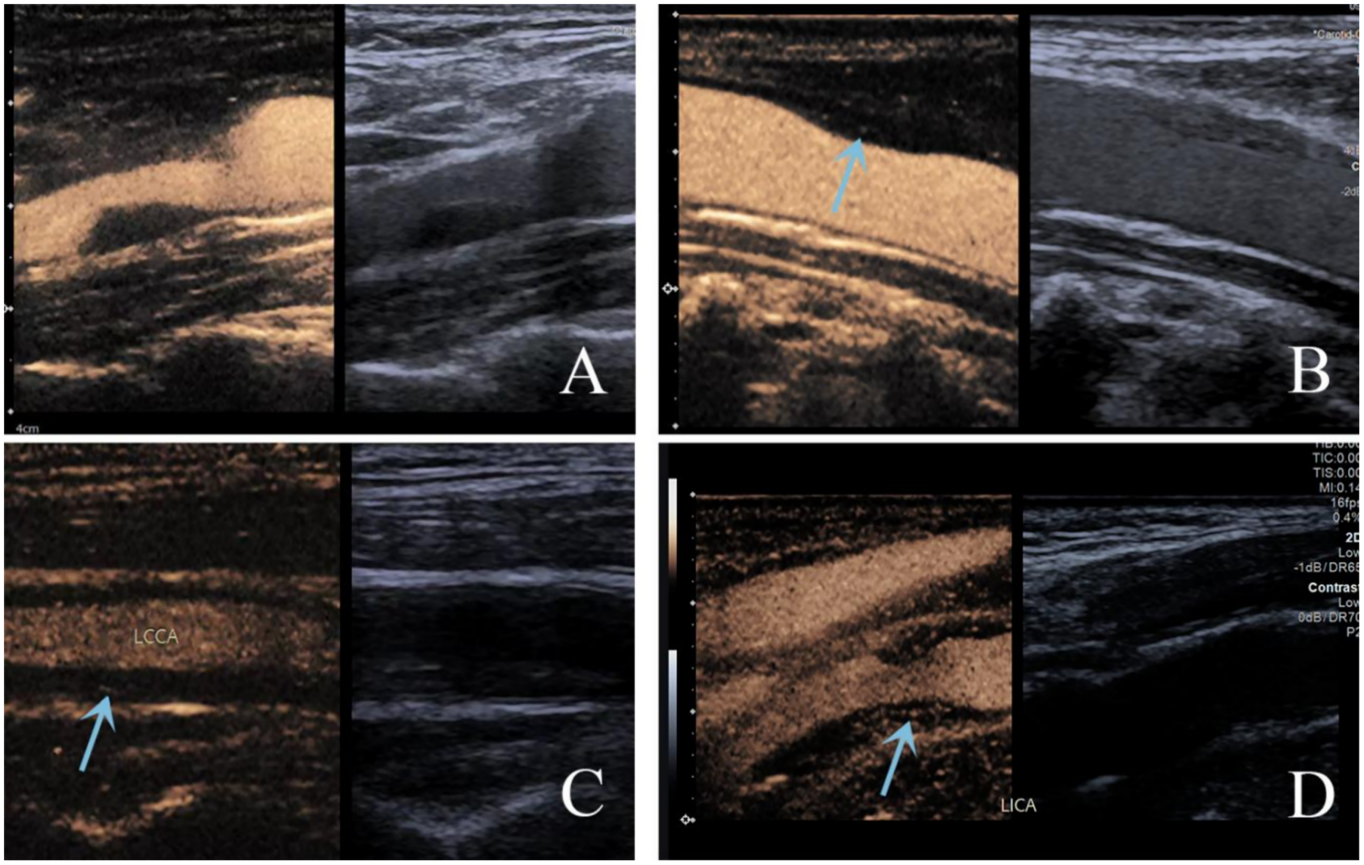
Standardized grading criteria for the degree of contrast enhancement within plaques on ultrasound: A Score 0: no intraplaque enhancement; B score 1: punctate intraplaque enhancement; C score 2: intermediate between scores 1–3, characterized punctate and 1–2 short linear of enhancement within the plaque; D score 3: linear intraplaque enhancement, penetrating or involving the majority of the plaque, orvisible signs of blood flow.
